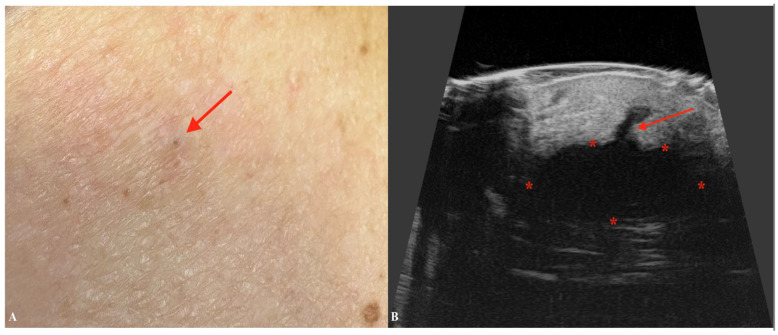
Macroscopic image of a cheek epidermoid cyst (A), with an arrow indicating the cyst orifice. The corresponding ultrasound image (B) displays the epidermoid cyst with a visible duct (arrow). Stars indicate the lesion margins.
